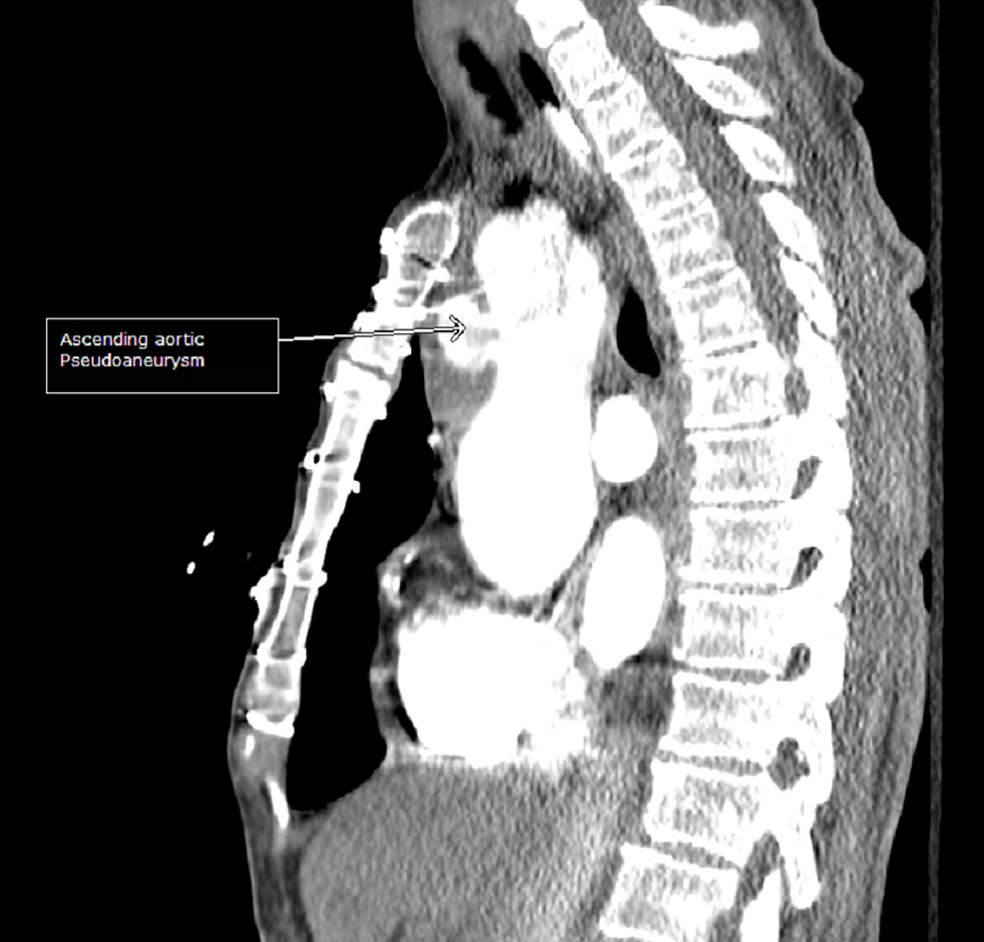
CT angiogram (sagittal view) demonstrating ascending aortic pseudoaneurysm.
